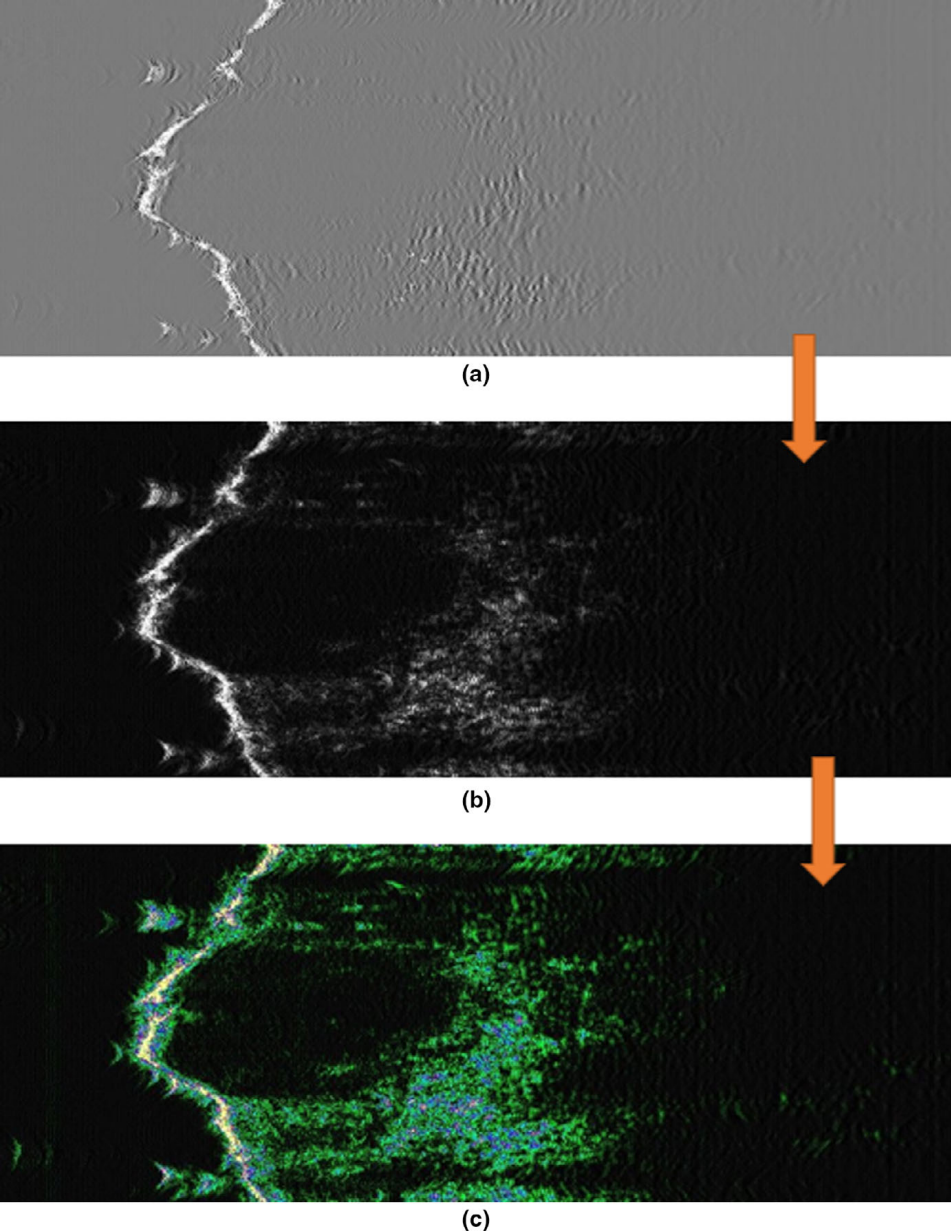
50 MHz sonogram used in this project (a) RF mode (b) B‐mode (c) customized color map.
